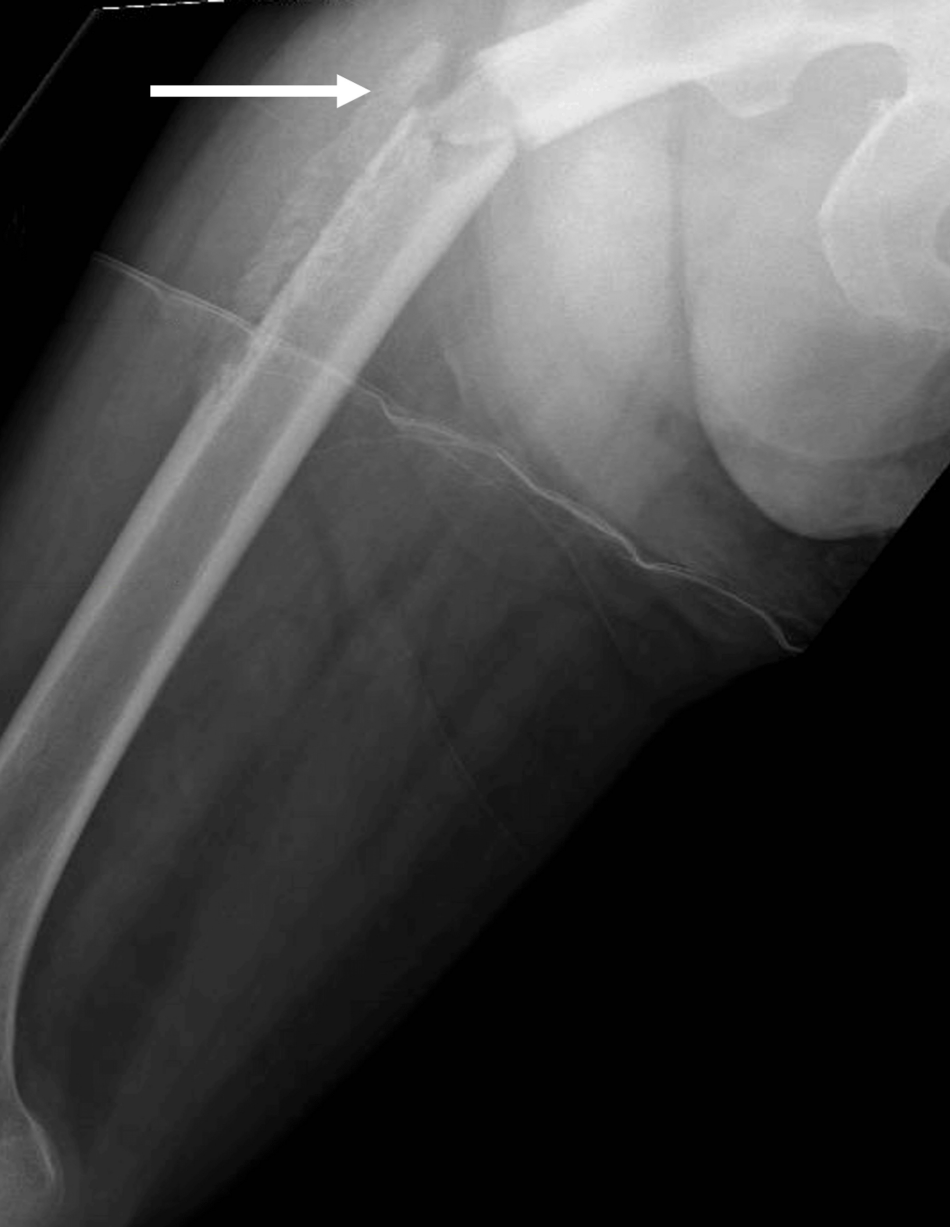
Displaced fracture (arrow) of proximal right femoral shaft on right femur radiograph
